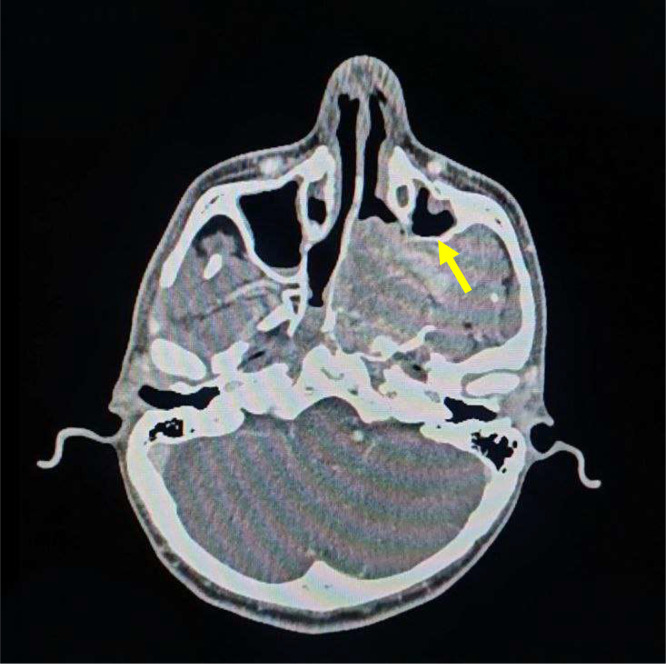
Axial noncontrast CT scan showing the pathognomonic Holman–Miller sign (antral sign), characterized by the anterior bowing of the posterior wall of the left maxillary sinus (arrow) due to the mass effect from the nasopharyngeal tumor.
